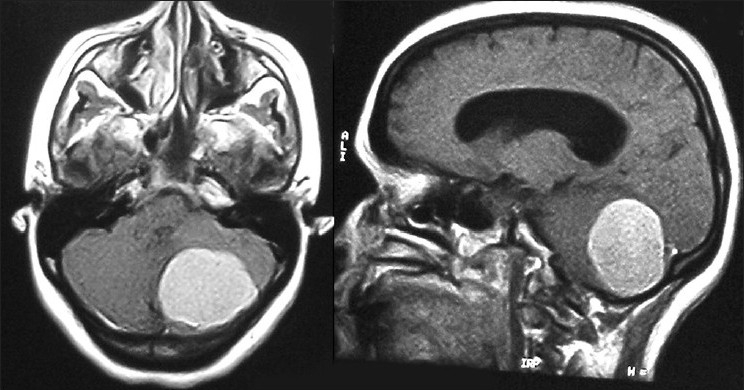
Preoperative MRI brain contrast T1W images showing large posterior fossa tumor on left side with mass effect and obstructive hydrocephalus
